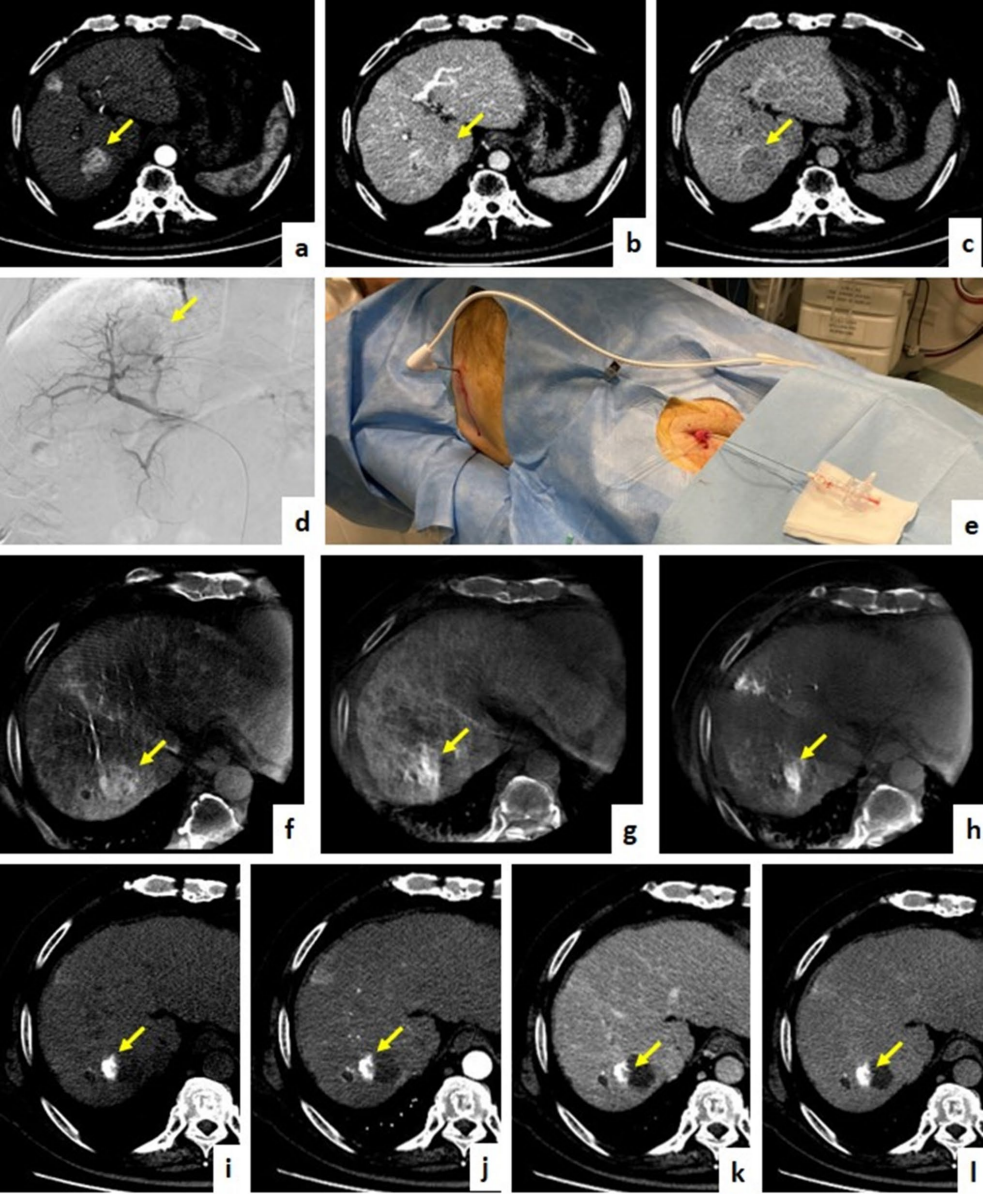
Seventy-four-years-old patient with presence of HCC lesion in the VII hepatic segment as shown in triphasic contrast enhanced CT pretreatment, arterial phase (a), venous phase (b), delayed phase (c). After initial angiographic study (d), single-session approach with MWA (e) followed by cTACE was performed. CBCT with intra-arterial contrast medium injection was performed before treatment (f) and after MWA (g), whereas CBCT without medium of contrast was performed after cTACE (h). Follow-up imaging with contrast enhanced CT shows no residual HCC and peri-lesional lipiodol distribution with central necrotic area, basal acquisition (i), arterial phase (j), venous phase (k), delayed phase (l)
